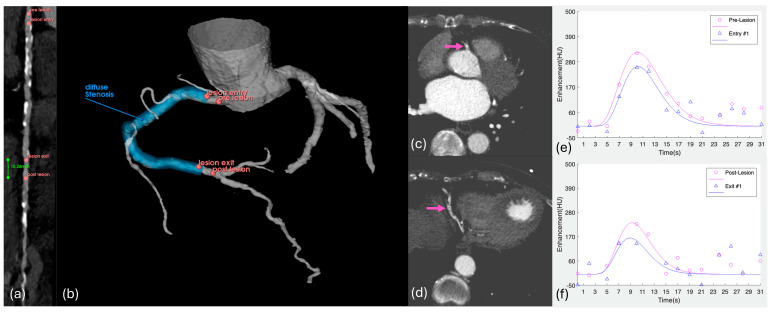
A 72-year-old female (patient 16 in Table 1) whose right coronary artery (RCA) had a long 75% diffuse stenosis that extended from the proximal to distal segment. The FFR value measured in the distal segment (coronary segment #3) with CT-DAI was 0.83 and agreed with that obtained using the invasive method (0.83). (a) Pre-lesion, lesion entry, lesion exit and post-lesion sampling ROIs shown in the straightened lumen view of the RCA. The calcified plaques below the post-lesion site were not included for the FFR analysis because the post-lesion ROI would have been placed in coronary segment #4. (b) Sampling ROIs shown in the three-dimensional image of the RCA. (c) Pre-lesion ROI (pink arrow) and (d) post-lesion ROI (pink arrow) shown in the axial CT myocardial perfusion image at one time point. (e,f) The plots show the raw data points sampled from the ROIs shown in (a–d) and the corresponding fitted coronary time-enhancement curves.
